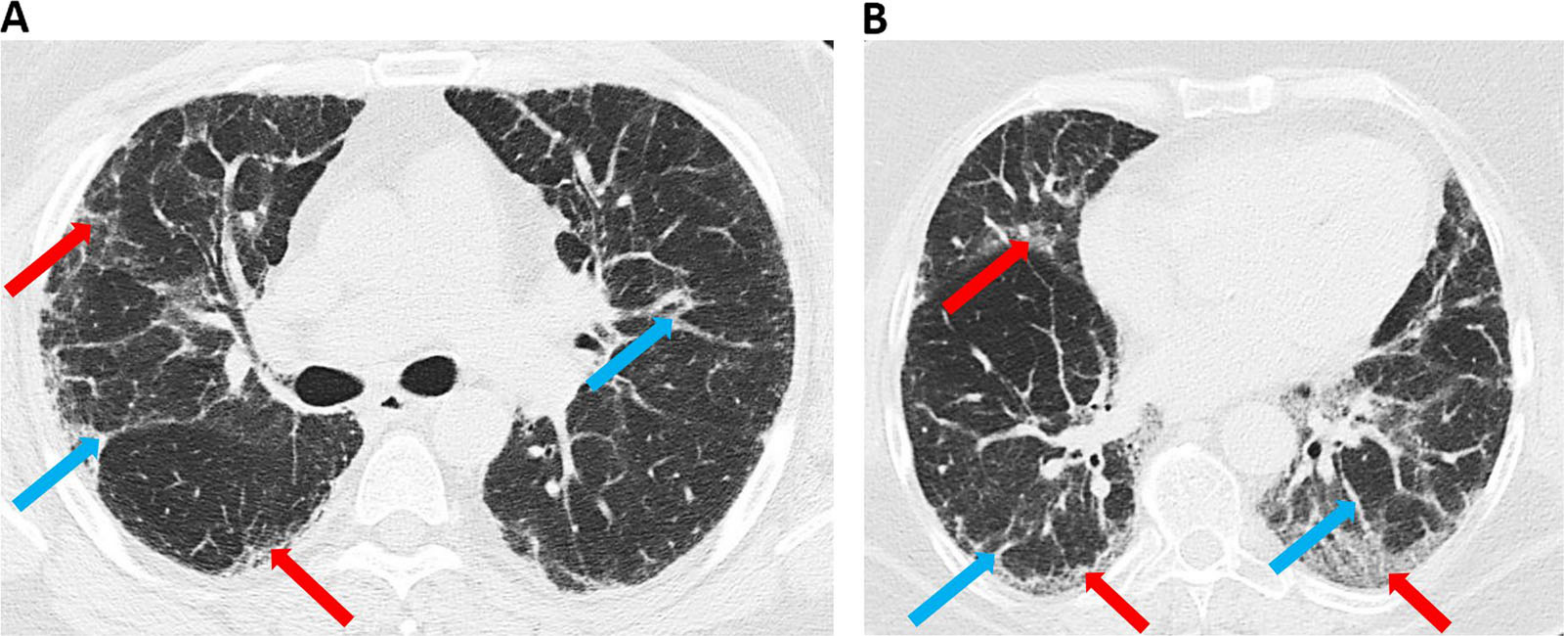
Chest high-resolution computed tomography findigs. Chest HRCTs (high-resolution computed tomography; transverse plane) of the proband (II-1) (a) and the proband´s mother (I-2) (b). Inter- and intralobular septal thickening and irregular reticular lines (blue arrows), ground glass opacities arrows, and crazy paving and traction bronchiectasis without honeycombing (red arrows) are shown
